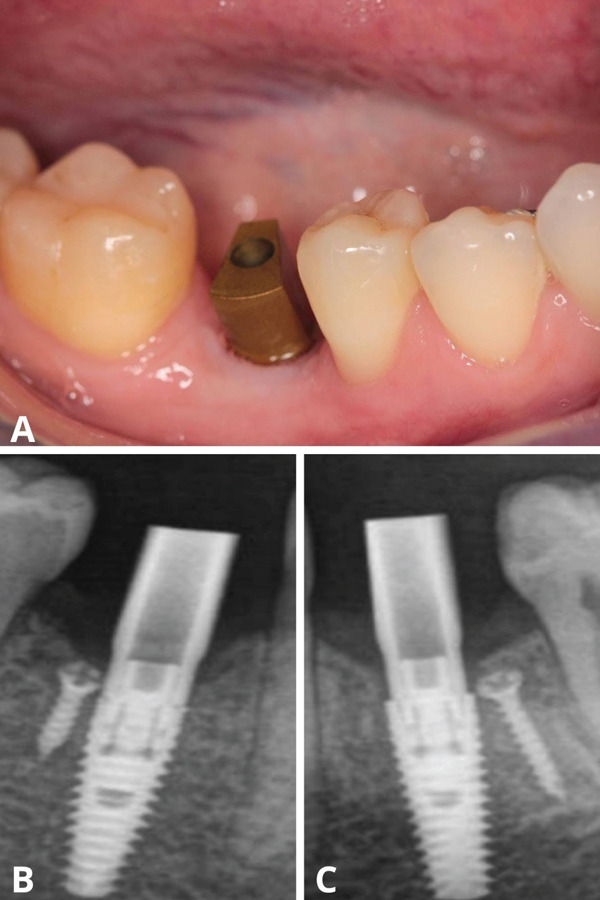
Digital impression for implant‐supported crowns. (A) Scan postscrewed onto the implant, (B) control radiograph—36, and (C) control radiograph—46.
